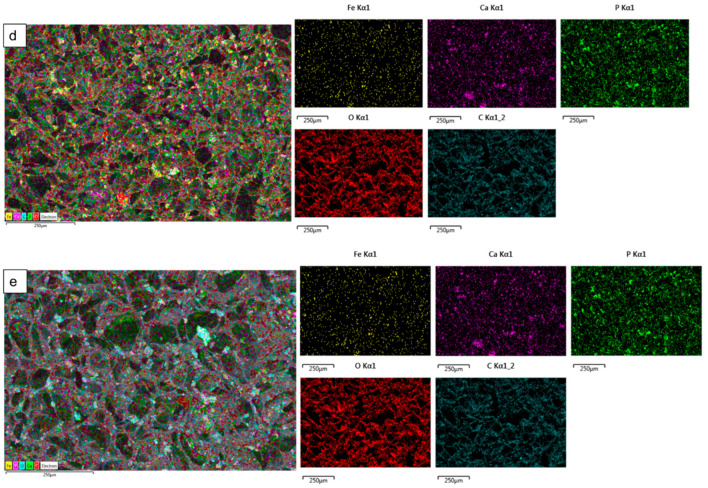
The Hitachi SU8230 SEM with a dispersive energy X-ray (EDX) detector was used to analyse the surface elemental characterization of freeze-dried chitosan scaffolds without Fe^3+^-DCPD mineral and with various Fe^3+^-DCPD mineral concentrations (20, 30, 40, and 50 (wt)%). Freeze-dried (a) Chitosan, (b) 20-Fe^3+^-DCPD, (c) 30-Fe^3+^-DCPD, (d) 40-Fe^3+^-DCPD, and (e) 50-Fe^3+^-DCPD scaffold. Each colour represents different types of elements in the sample.
